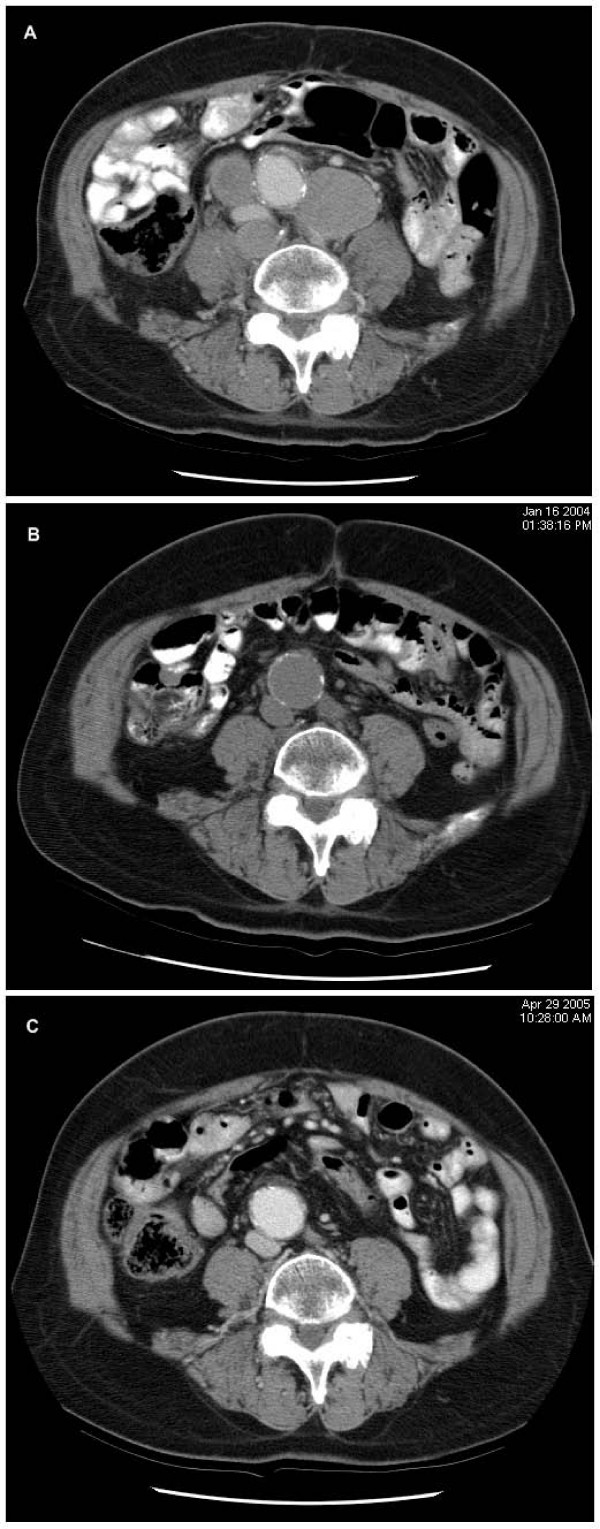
Radiographic response to Bicalutamide withdrawal. Panel A depicts computed tomography scan of abdomen and pelvis with significant periaortic and retroperitoneal adenopathy about 2 months after cessation of bicalutamide. Panel B depicts dramatic egression of adenopathy in response to bicalutamide withdrawal after 8 months. Panel C depicts durable response 20 months after bicalutamide withdrawal.
